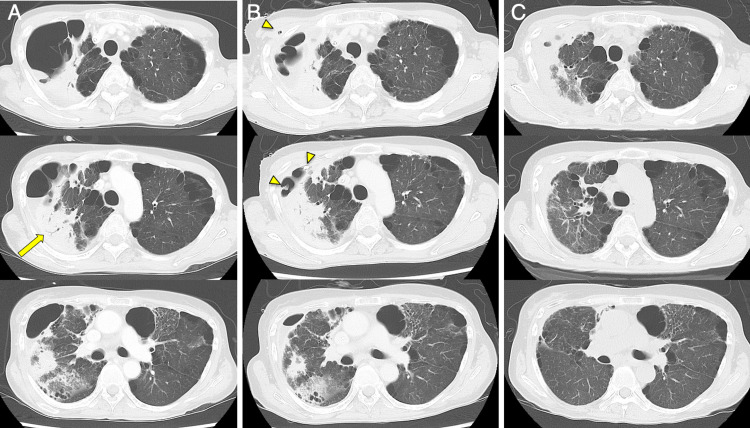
Chest CT images of the patient. Chest CT images (A) on admission to Hamanomachi Hospital, (B) during percutaneous drainage on day 50, (C) before discharge from Hamanomachi Hospital on day 88. An arrow indicates the lesion where trans-bronchial lung biopsy was performed. Arrowheads indicate a percutaneous drainage tube inserted into the lung bulla.
